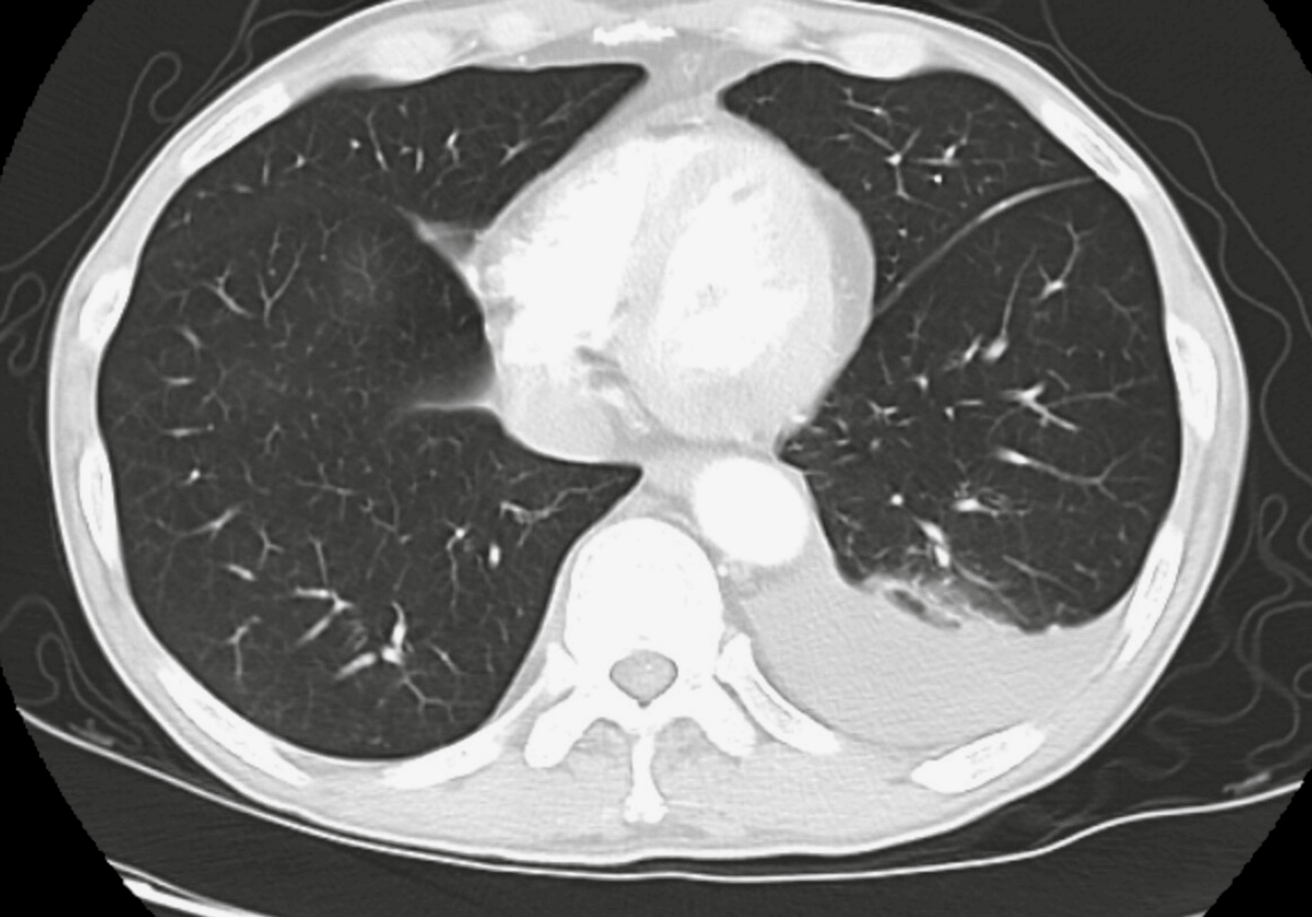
CT chest showing a large, complicated, left-sided pleural effusion
